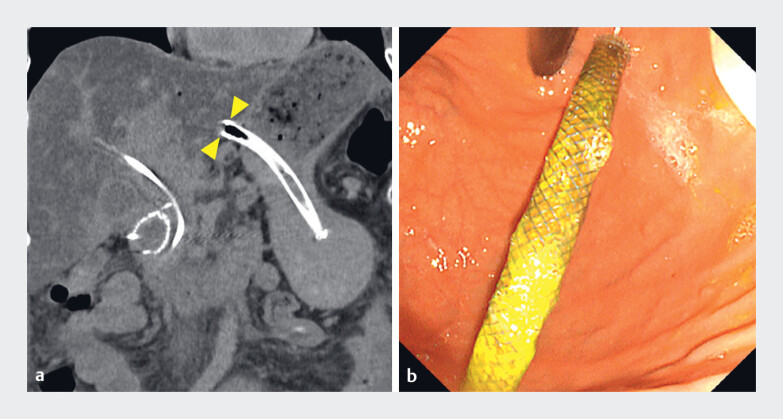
Migration of the covered metal stent toward the gastric lumen after its placement during endoscopic ultrasound-guided hepaticogastrostomy.
